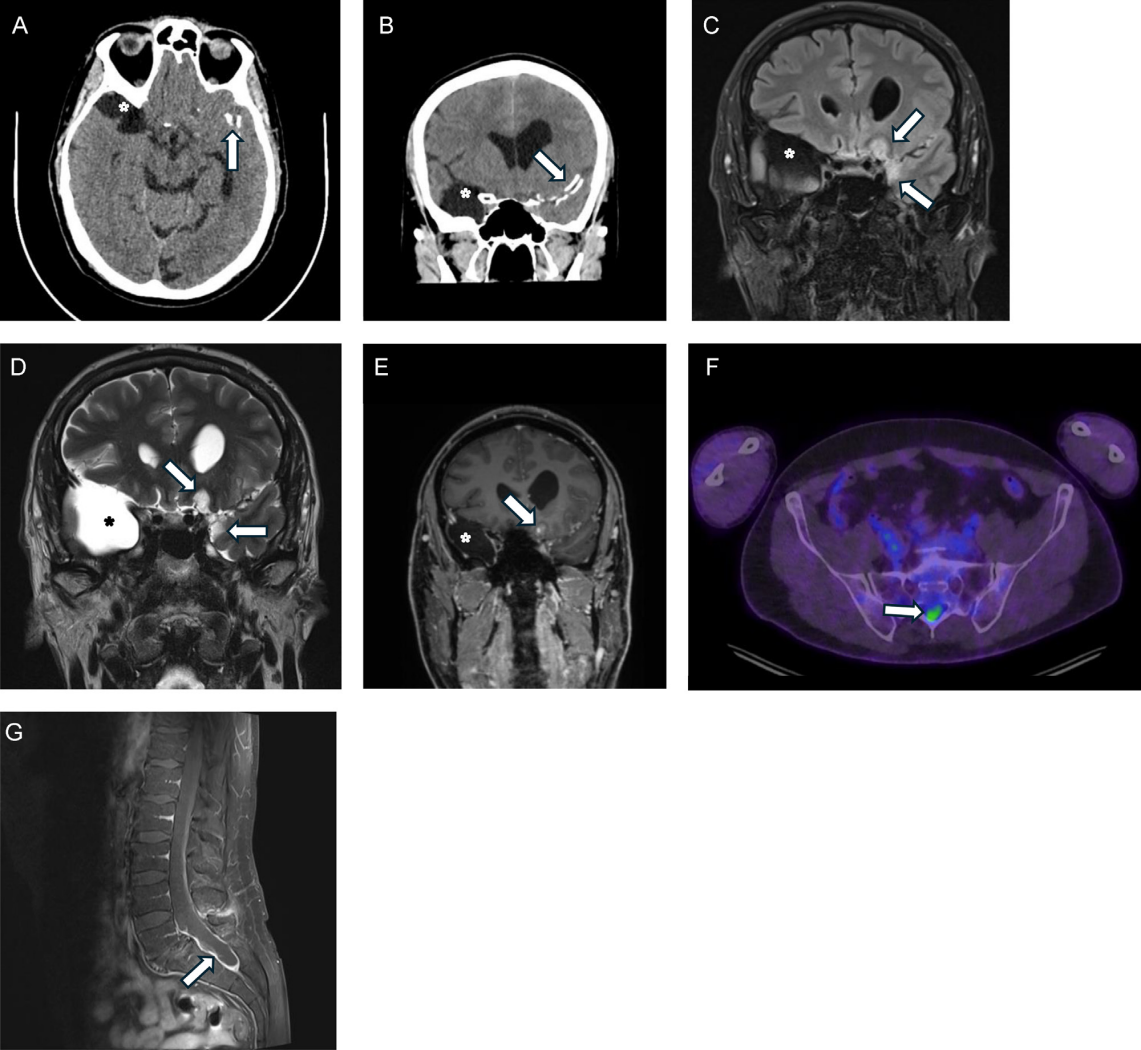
CT head axial (A) and coronal plane (B) sections demonstrating calcification along the left sylvian fissure (arrows). Incidental right temporal pole arachnoid cyst (asterisks) on MRI and CT (A-E). Coronal FLAIR (c) and T2 weighted MRI (D) show heterogenous hyperintensity involving medial left temporal and frontal lobes (arrows), with widening and displacement of the left olfactory sulcus. There is patchy leptomeningeal enhancement (arrow) after intravenous contrast on coronal T1-weighted section (E). An axial plane section from the FDG-PET study at the level of the pelvis (F) demonstrates abnormal tracer uptake within the sacral spinal canal (arrow). Sagittal plane fat-suppressed T1 MRI acquired after IV contrast (G) demonstrates distension, diffuse thickening and hyperintensity of the sacral thecal sac (arrow).
